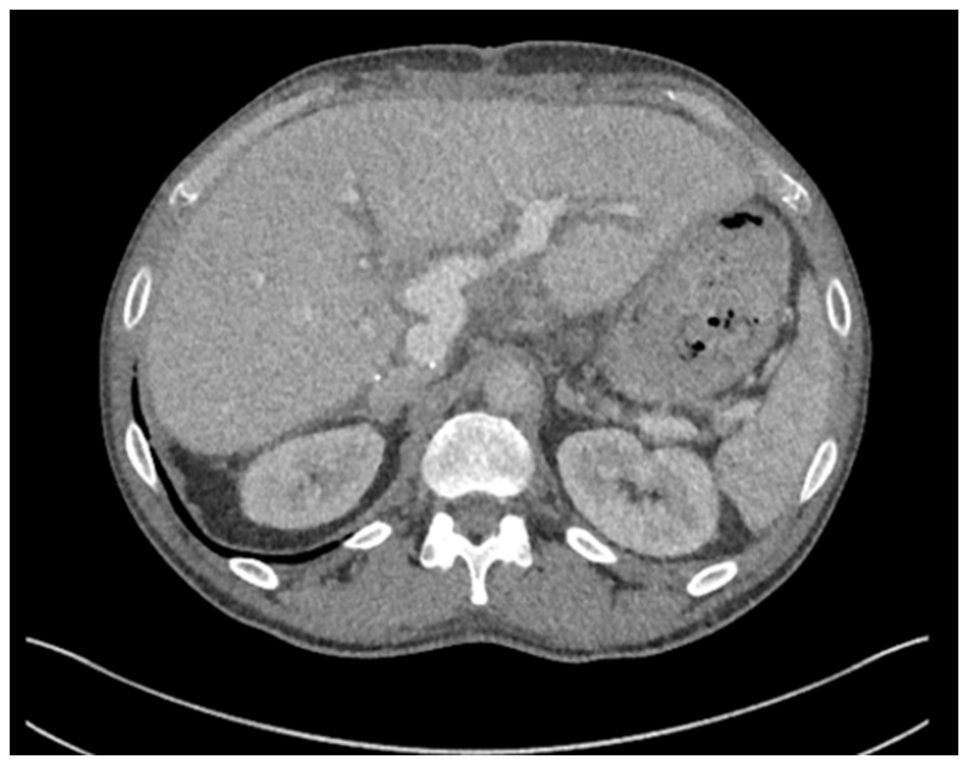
Orthotopic liver transplantation using a whole-liver graft from a brain-dead donor in 2019. The figure depicts a post-operative computed tomography scan performed in January, 2020, prior to discharge, after a long recovery from surgery.
